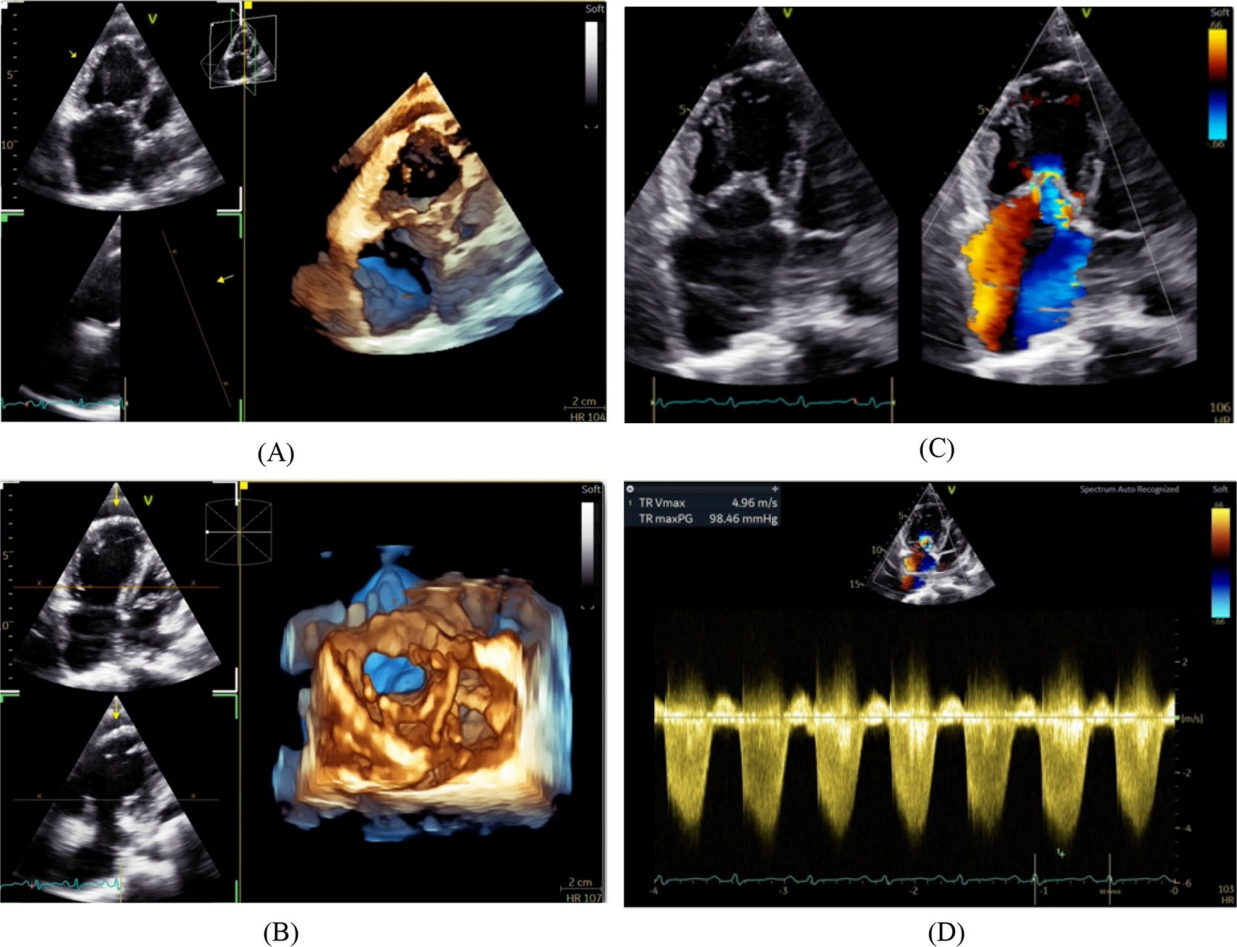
A, B 3D-TEE: Significant thickening and calcification of all tricuspid valve leaflets, with a partial prolapse suspected in the posterior leaflet; C 2D-TTE: The tricuspid valve (TV) is experiencing a significant backflow of blood, as observed using color Doppler imaging. Wall-impinging jet accounts for more than 50% of the right atrium's volume. The vena contracta width measured 0.7 cm, and the TR was predominant in the right atrium, right ventricle, and right atrium exhibited enlargement. D 2D-TTE: Continuous wave The Doppler analysis of the TV revealed a jet with a pansystolic pattern, characterized by a dense, semi-triangular form that reached its maximum intensity early. The peak velocity of the jet was measured at 4.9 m/s
